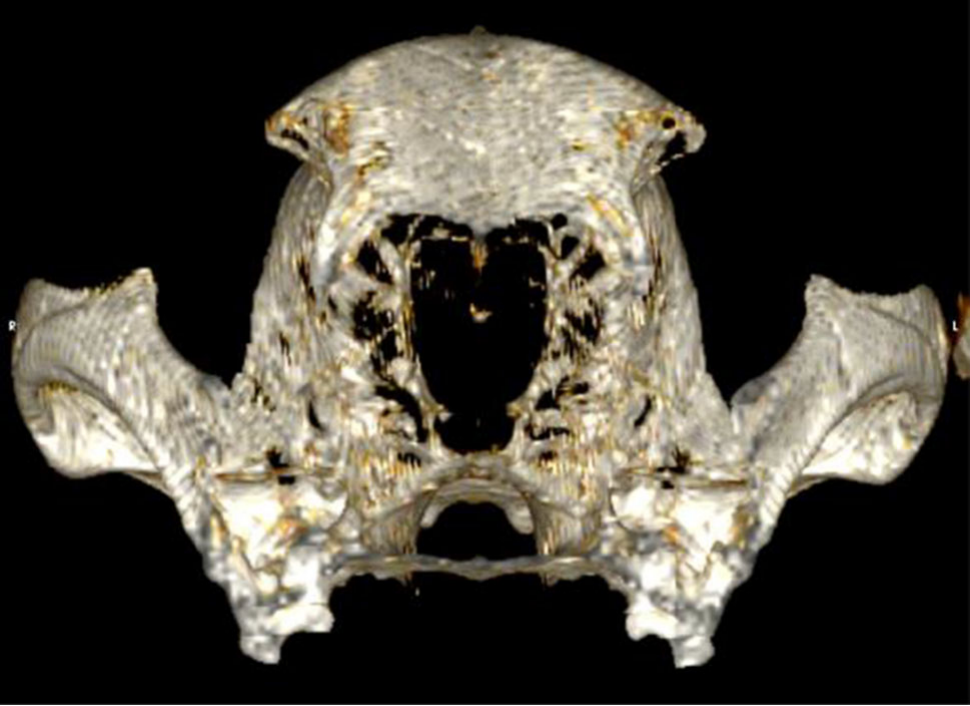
A 3D CT model reconstruction of a wolf skull in the OsiriX MD software program. Rostral view
